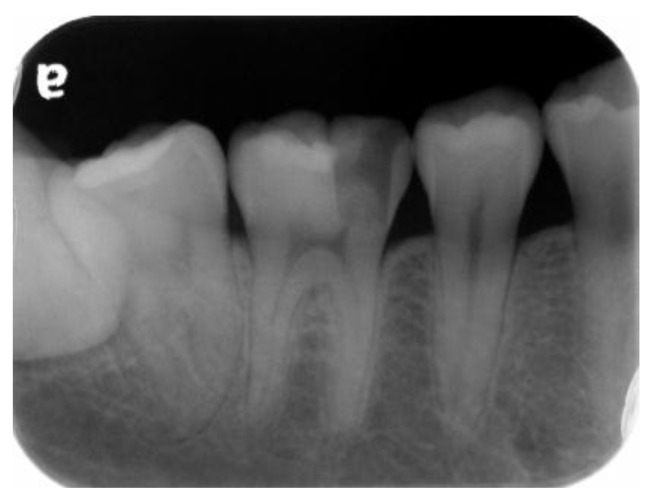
Pre-operative periapical radiograph shows caries in proximity to the pulp horn.
